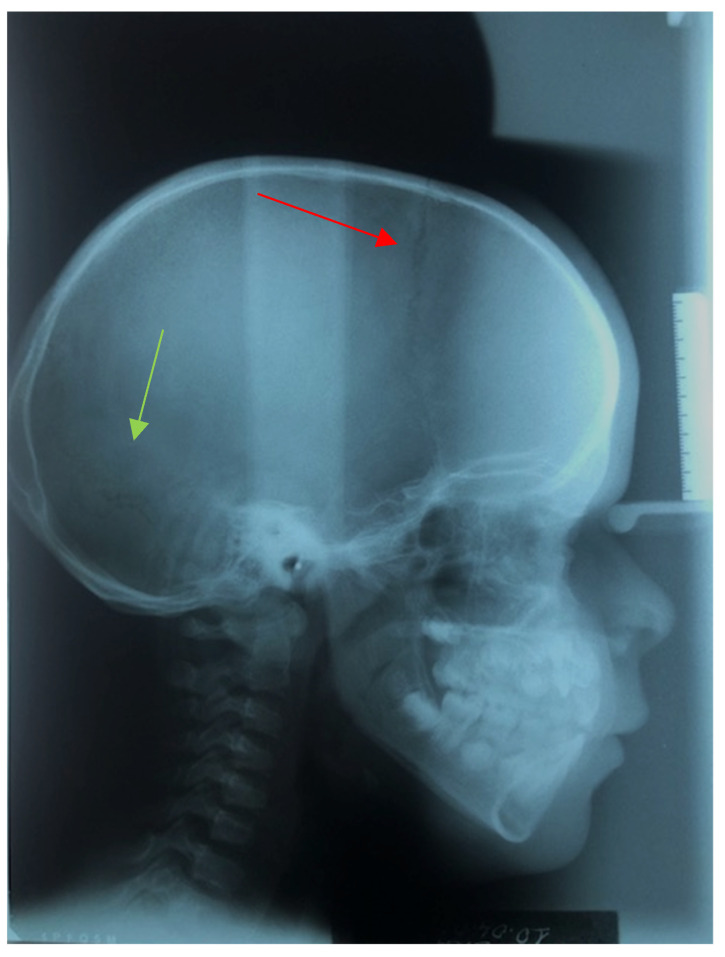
Initial 10 April 2008 teleradiography X-ray sagittal plane (10-year-old): evidence of delayed closure of cranial suture (arrows).
